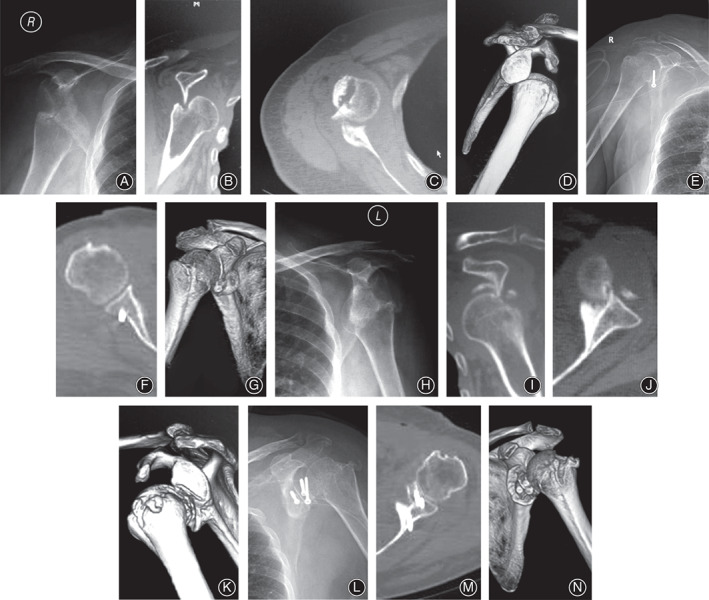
A 67 years old woman with bilateral chronic anterior dislocation, right anterior shoulder dislocation more than 2 months, left anterior shoulder dislocation more than 8 months (No. 17 patient). (A): Preoperative anteroposterior radiograph showing a chronic anterior dislocation. (B‐D): Preoperative CT scans showing chronic anterior dislocation with Hill‐Sachs lesion. Postoperative anteroposterior radiograph showing right shoulder reduction with Bristow procedure. (E‐G): Postoperative CT scans showed right shoulder was in restoration. (H): Preoperative anteroposterior radiograph showing a chronic anterior dislocation. (I‐K): Preoperative CT scans showing chronic anterior dislocation with Hill‐Sachs lesion and pseudoarthrosis formation. (L): Postoperative anteroposterior radiograph showing left shoulder reduction with Latarjet procedure and iliac graft block. (M, N): Postoperative CT scans showed left shoulder was in restoration and the location of screws was good.
